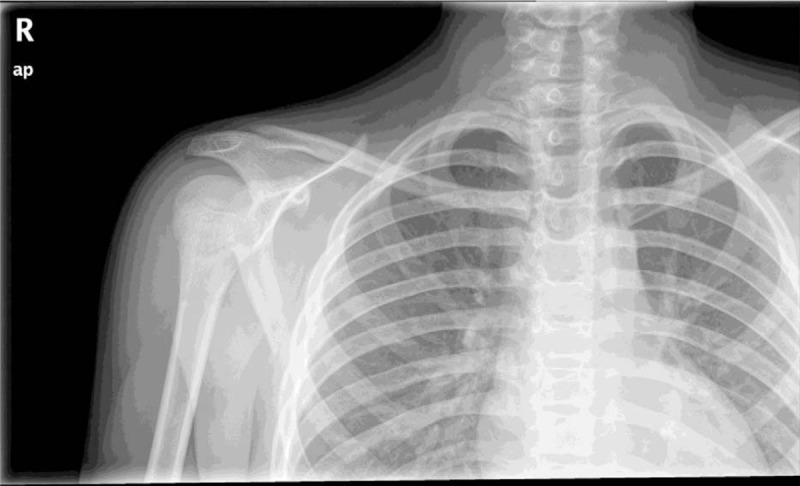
Anteroposterior thoracic X-rays.
